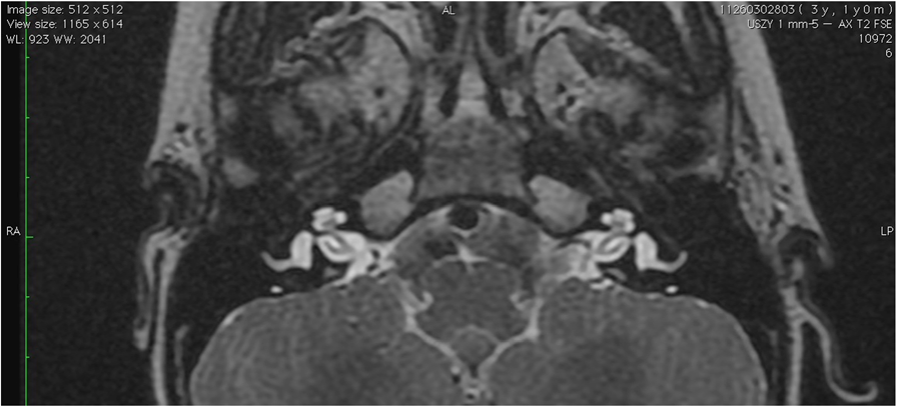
MRI T2-weighted axial image of the ear before the first CI surgery at 1 year of age showing no middle ear pathology
